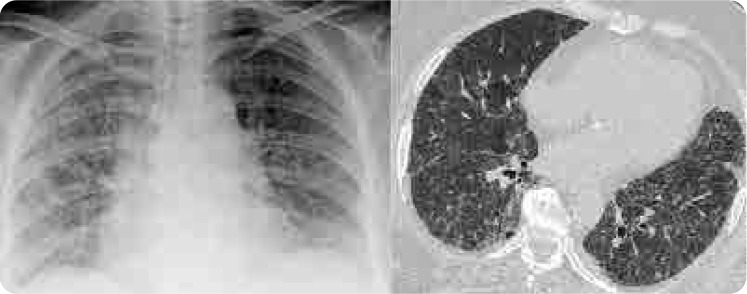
Patient's X-ray and HRCT images at presentation: Chest X-ray showed bilateral infiltrates, HRCT revealed diffuse ground-glass and reticular opacities.
